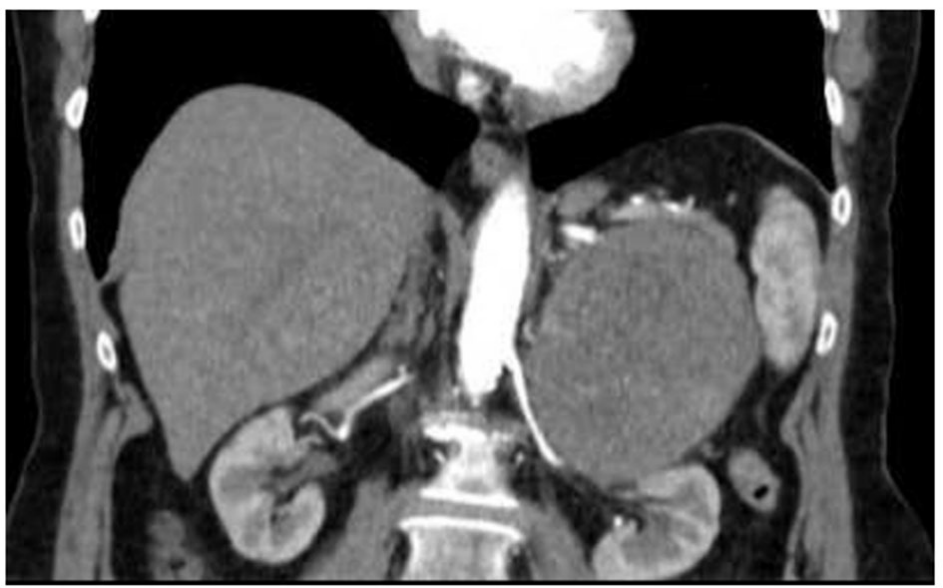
CT-scan of the abdomen. Coronal-view CT scan of the abdomen reveals a large abdominal mass measuring 10 × 8 × 10 cm without lymphadenopathy.
